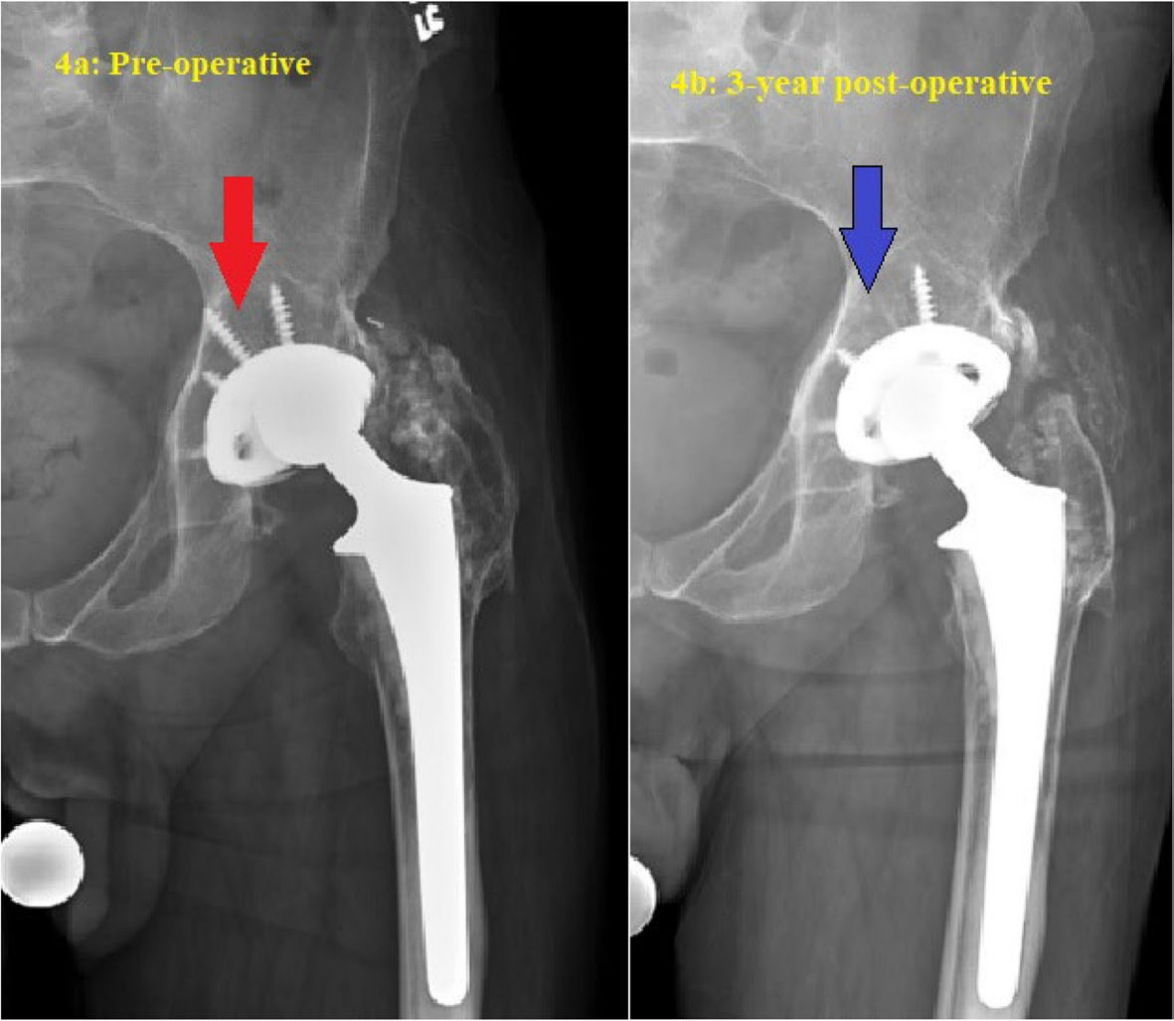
Serial imaging of an 80-year-old male following isolated liner and head exchange for retro-acetabular osteolysis secondary to polyethylene wear. The well-fixed cup and stem were retained. A single screw was removed to access the retro-acetabular region for grafting with demineralized bone matrix using a vinyl urinary catheter and syringe. a Pre-operative radiograph showing retro-acetabular osteolysis around the screw (red arrows); b Three-year post-operative radiograph demonstrating the incorporation of demineralized bone matrix (blue arrows) in the previous osteolytic lesion
